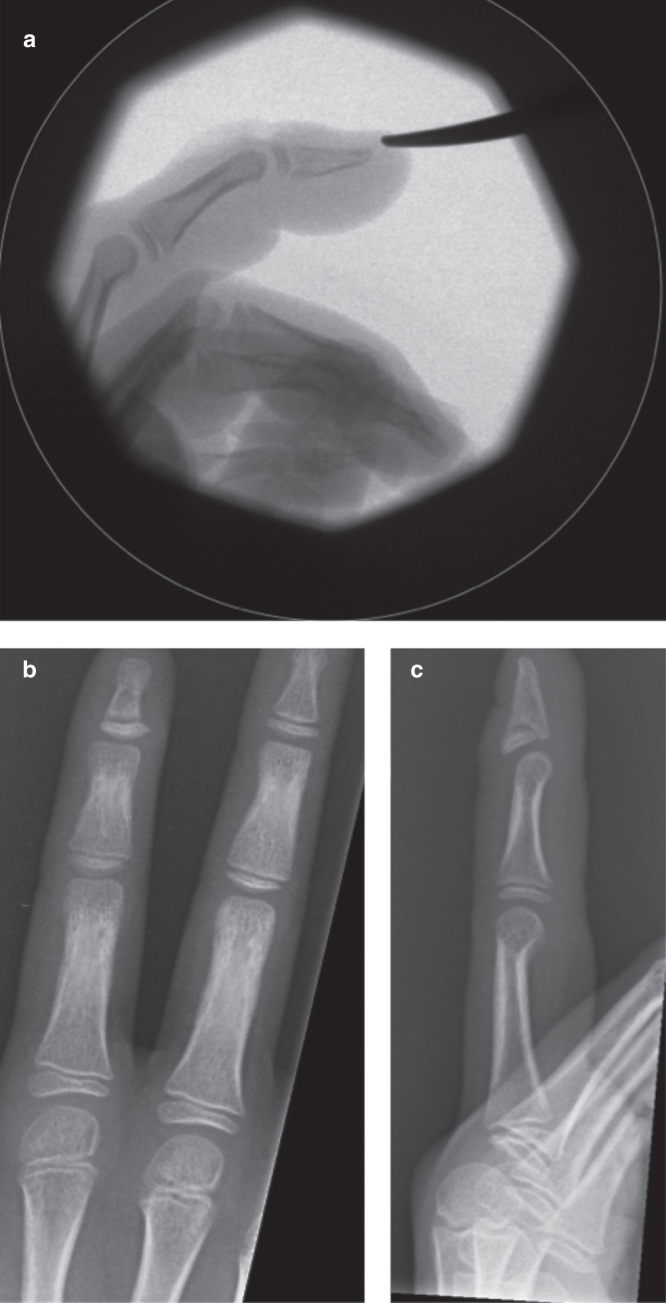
Intra-operative radiograph demonstrating reduced epiphysis (*a*). Post-operative radiograph at 6 weeks indicating subtle displacement of the epiphysis (*b and c*).
